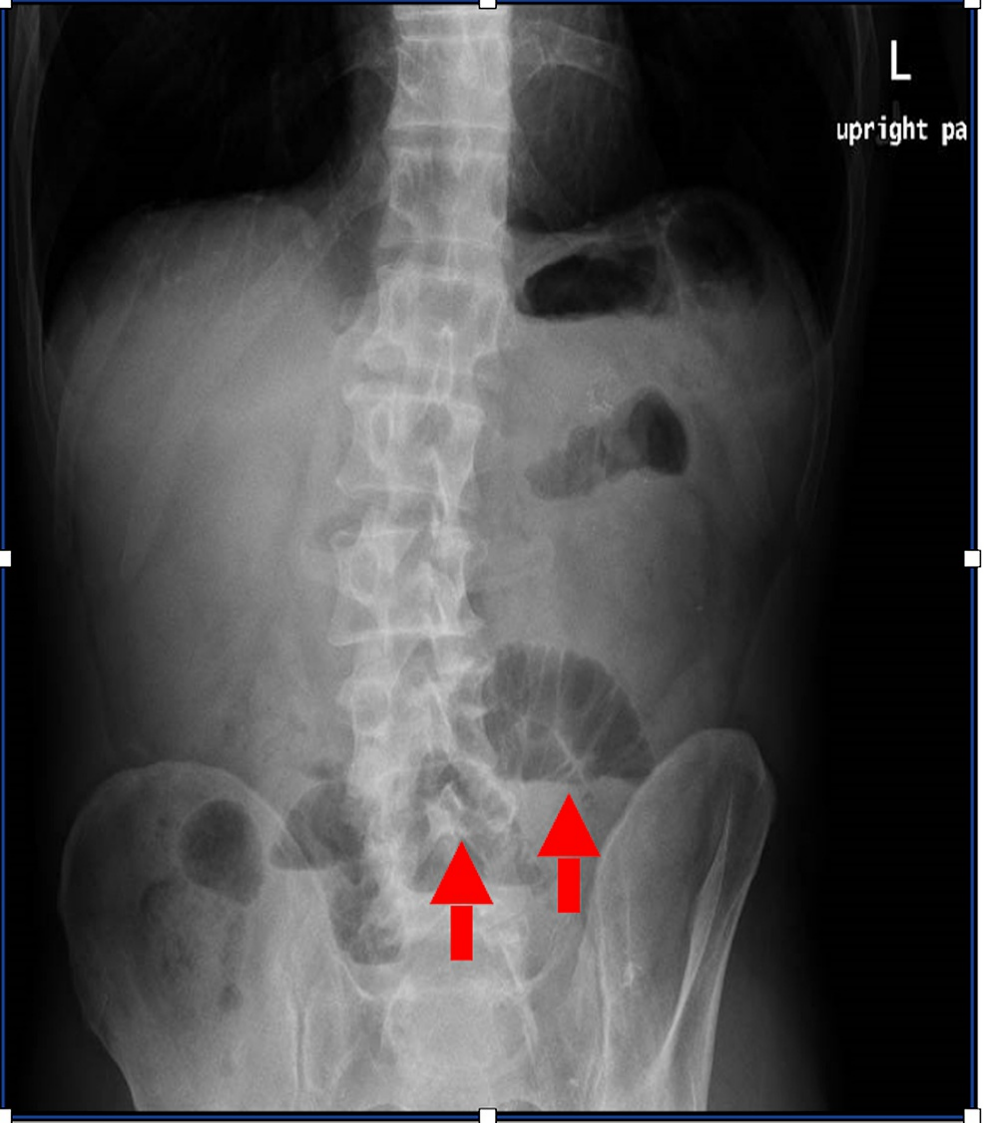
Upright abdominal X-ray Arrows indicate dilated small bowel loops that suggest evolving small bowel obstruction
